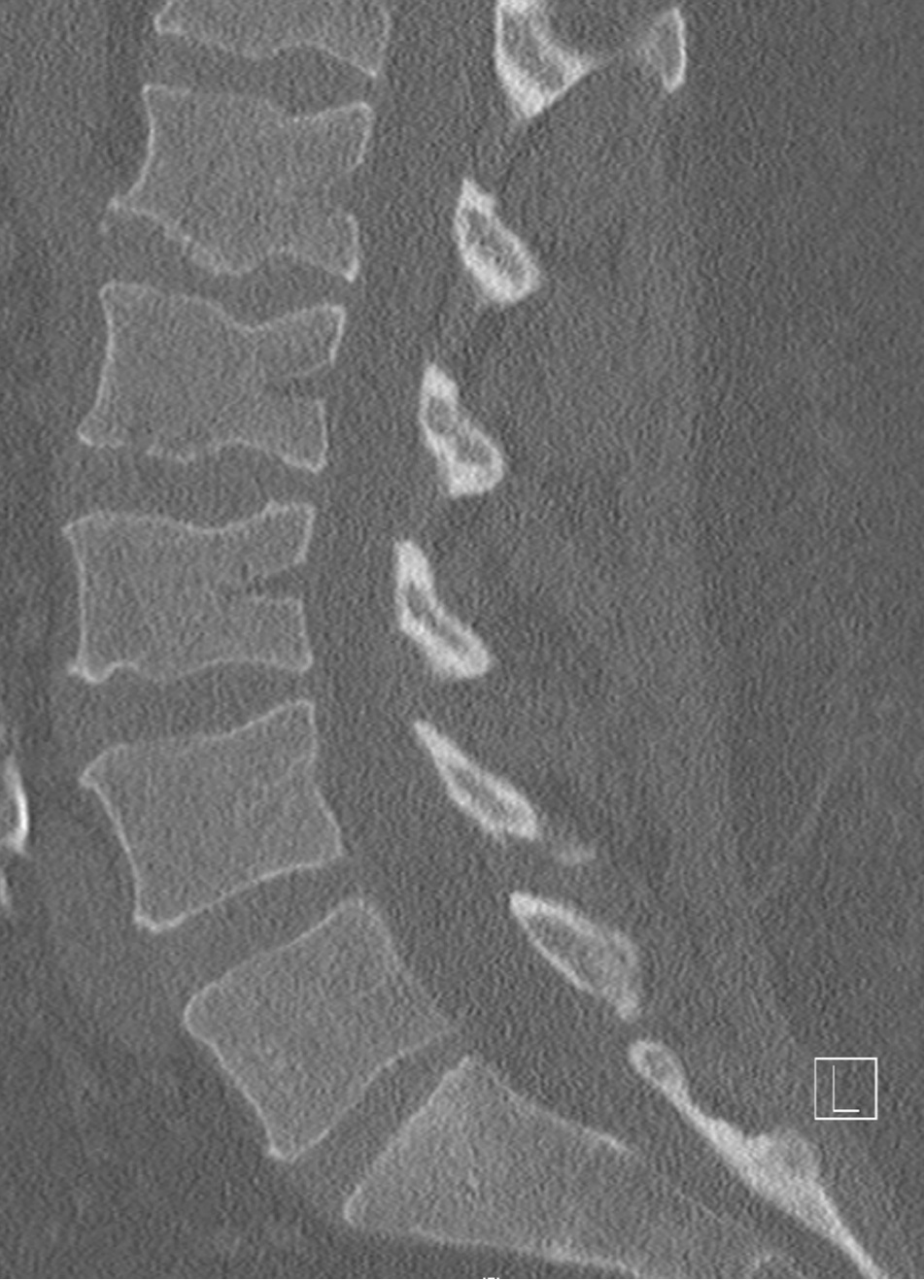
Initial CT revealing subtle irregularity of inferior endplate of L2.
